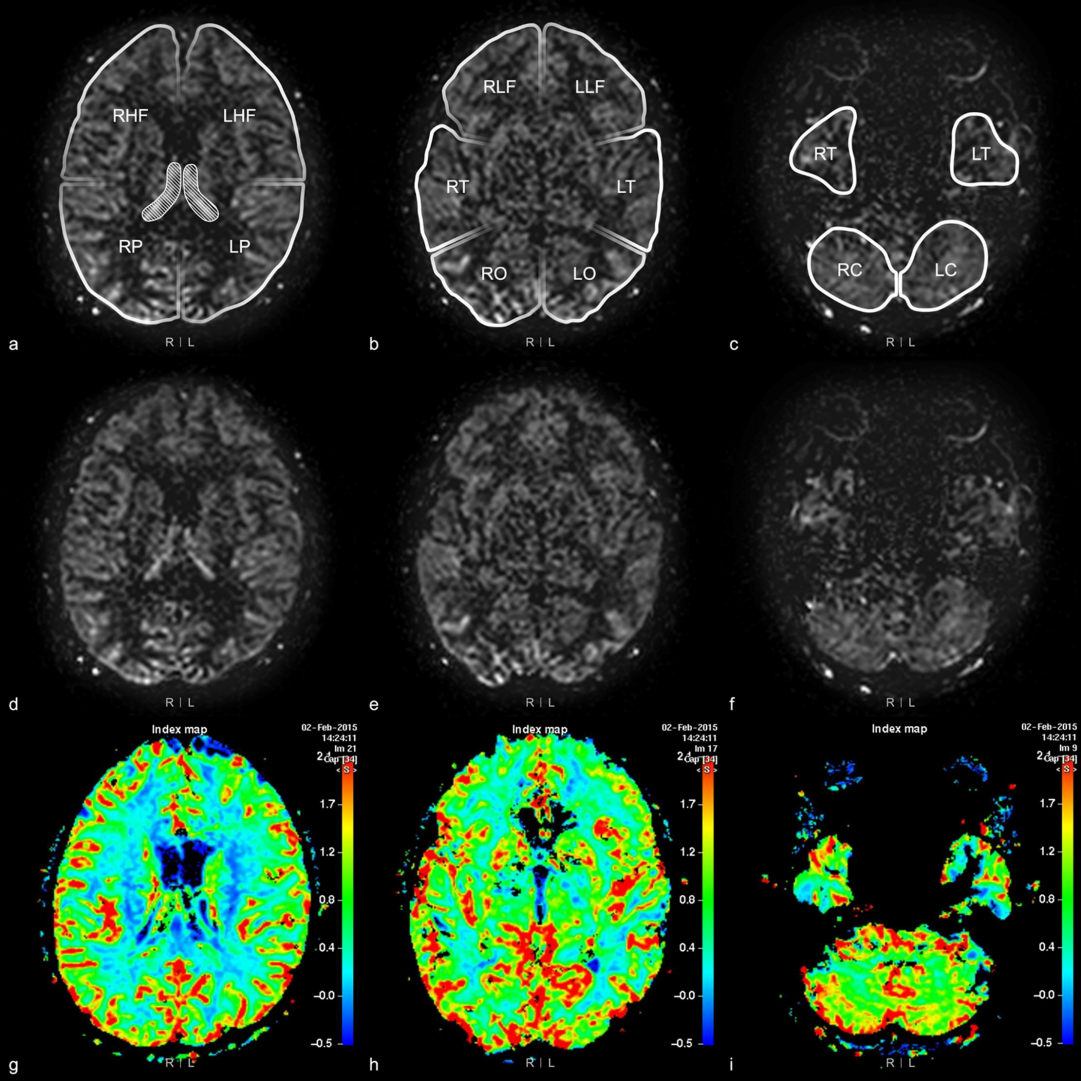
12 areas of bilateral cerebral and cerebellar hemispheres. Brain MRI of a 76-year-old woman with dizziness. 12 areas on arterial spin labeling images are shown in figures (a), (b), and (c). Figures (d), (e), and (f) are ASL images and figures (g), (h), and (i) are cerebral blood flow map. Figures (a), (d), and (g) show the most superior portion of the bilateral choroid plexus (regions marked by oblique lines in figure (a)). The image slices above the landmark, the choroid plexus in the trigone, are divided into right and left high frontal (RHF, LHF) and parietal (RP, LP) areas, and the lower image slices are divided into the other eight areas; the low frontal (RLF, LLF), temporal (RT, LT), occipital (RO, LO), and cerebellar (RC, LC) areas on both sides.
